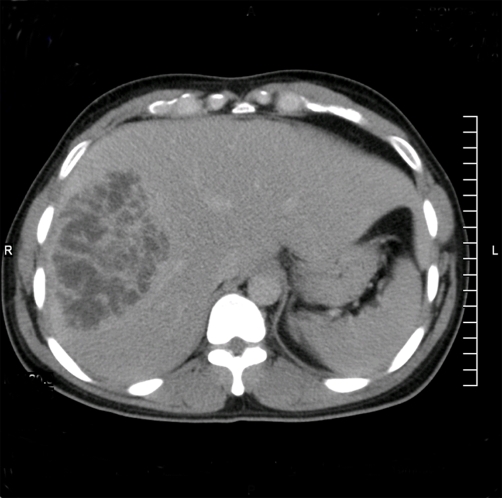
Computed Tomography scan of a 29 year old man with Klebsiella pneumoniae liver abscess. Abdominal Computed Tomography scan showing a 10 cm diameter Klebsiella related abscess. Notice, as a distinctive feature, the hypodense lesion with internal septa and irregular margins.
